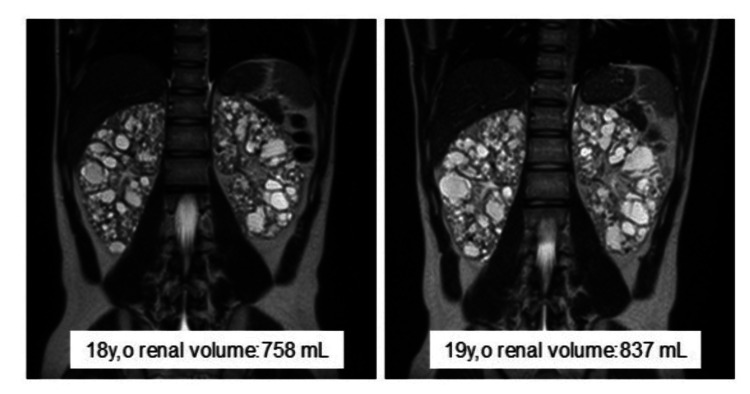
Abdominal MRI of the patient His kidney volume had increased from 758 mL to 857 mL over the course of a year MRI: magnetic resonance imaging
